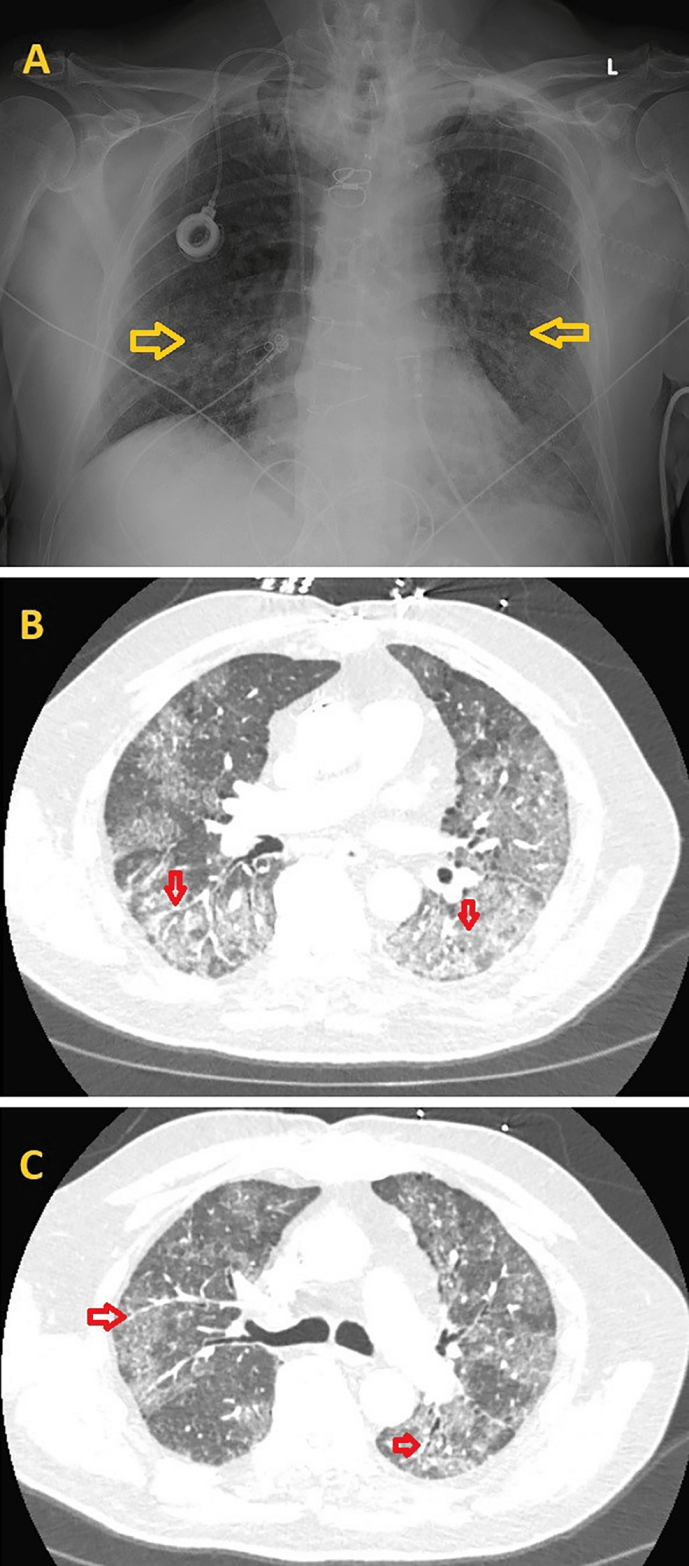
Initial chest X-ray (CXR) and computed tomography pulmonary angiogram (CTPA) findings 2A: CXR showing the prominence of interstitial markings (yellow arrows) predominantly in the mid and lower lung zones, suggestive of pulmonary edema or pneumonitis. 2B, 2C: CTPA showing bilateral ground glass airspace opacities with septal thickening (red arrows)
